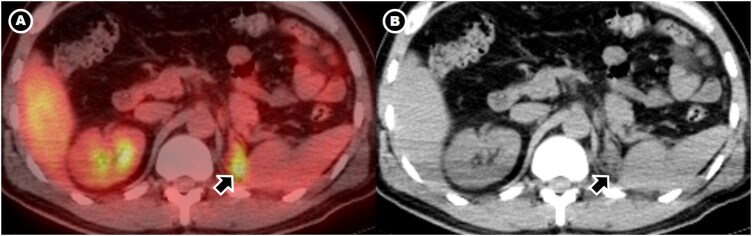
PET/CT workup demonstrating focal IgG4-related activity. Axial fused PET/CT (A) and non-contrast CT (B) through the surgical bed after IV administration of ^18^F-FDG, six weeks after left radical nephrectomy, show a moderately hypermetabolic soft-tissue focus (arrows; SUVmax 4.2) between the left diaphragmatic crus and spleen in the nephrectomy bed, consistent with active IgG4-related disease. No additional hypermetabolic foci suggestive of systemic IgG4-RD or metastatic RCC are identified, including in the contralateral kidney and central retroperitoneum.
